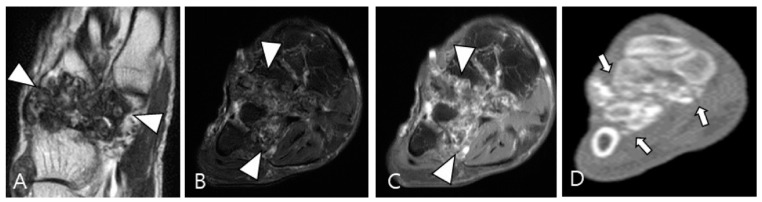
A 54-year-old male with synovial chondromatosis in the foot. (A) Coronal T2WI demonstrates diffusely low SI of synovial thickening at the Lisfranc or Chopart joints (arrowheads). (B) Axial fat-suppressed T2WI reveals infiltrative soft-tissue extension into the surrounding bone and muscles (arrowheads). (C) Axial contrast-enhanced fat-suppressed T1WI shows minimal peripheral enhancement (arrowheads). (D) Axial CT reveals multiple small conglomerated calcifications in involved tarsometatarsal joints (arrows) with bony erosion.
